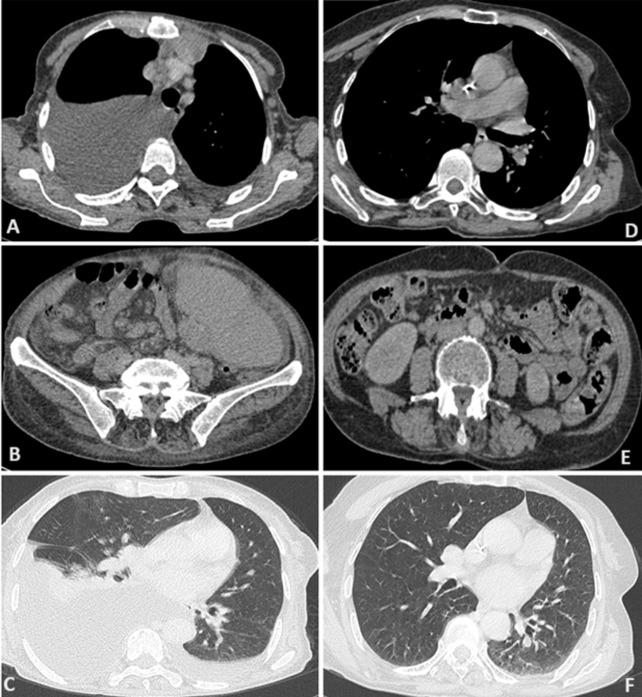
computed tomography scan of patients with stage VI mantle cell lymphoma before (A, B, C) and after (D, E, F) chemotherapy, demonstrating regression of tumor burden evident across thoracic, abdominal, and pelvic regions
